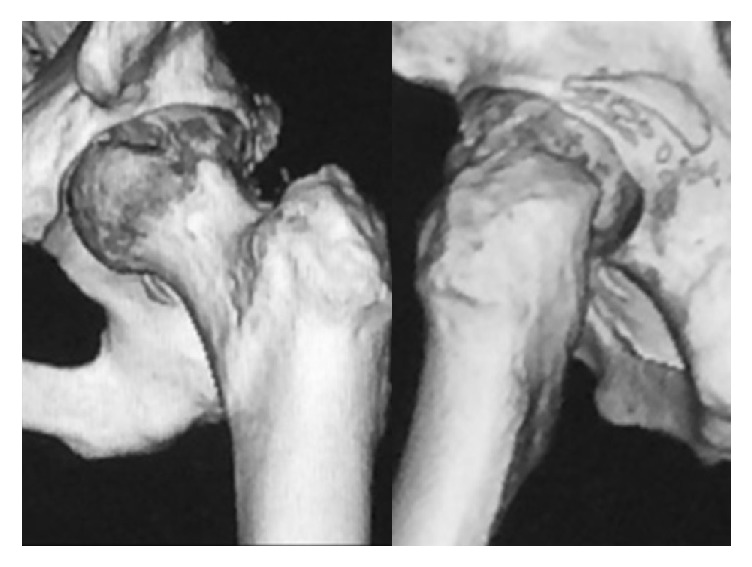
Computed tomography of the left hip joint after reduction.
